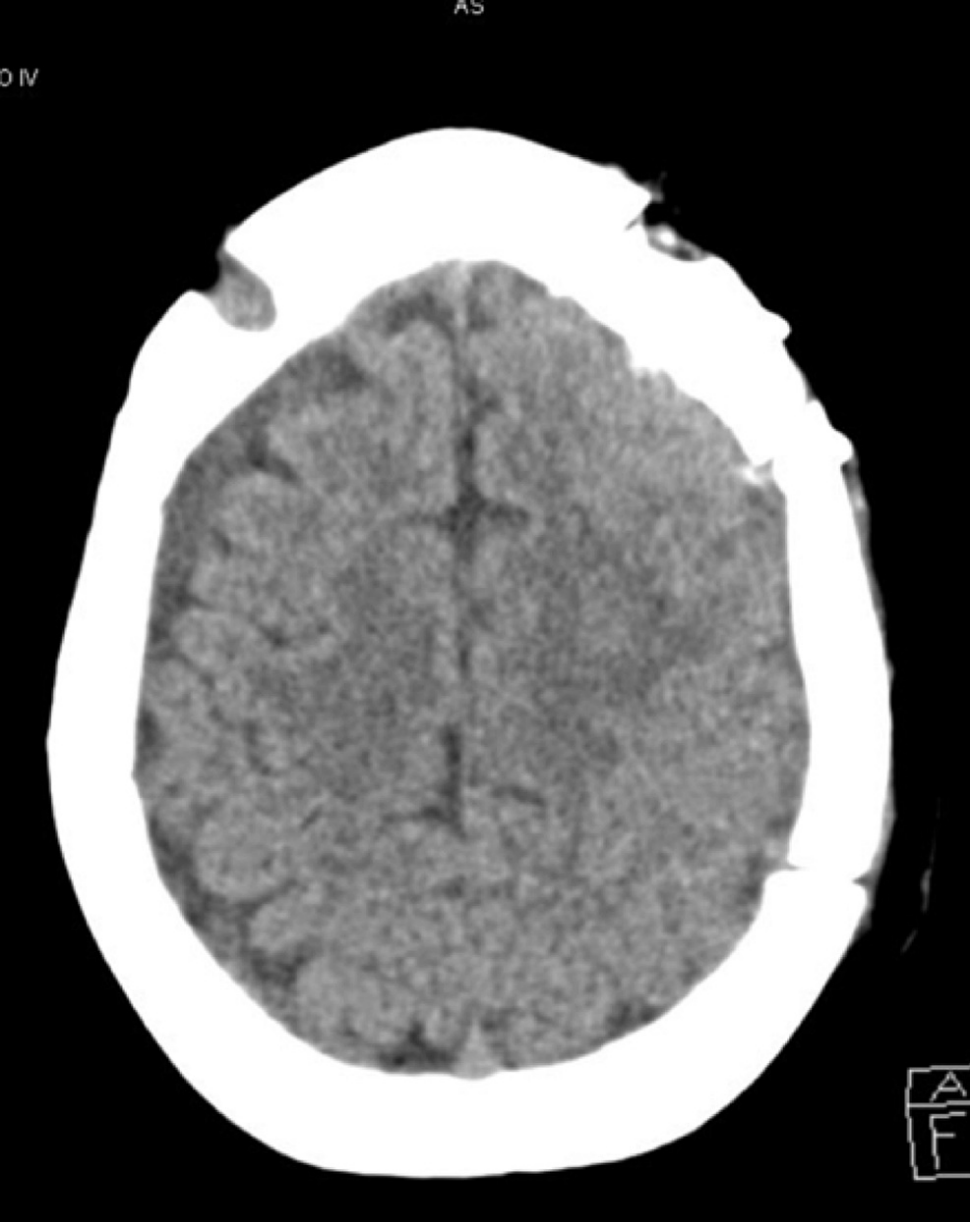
Brain CT. Longitudinal section: resolution of left subdural hematoma after burr hole surgery.
